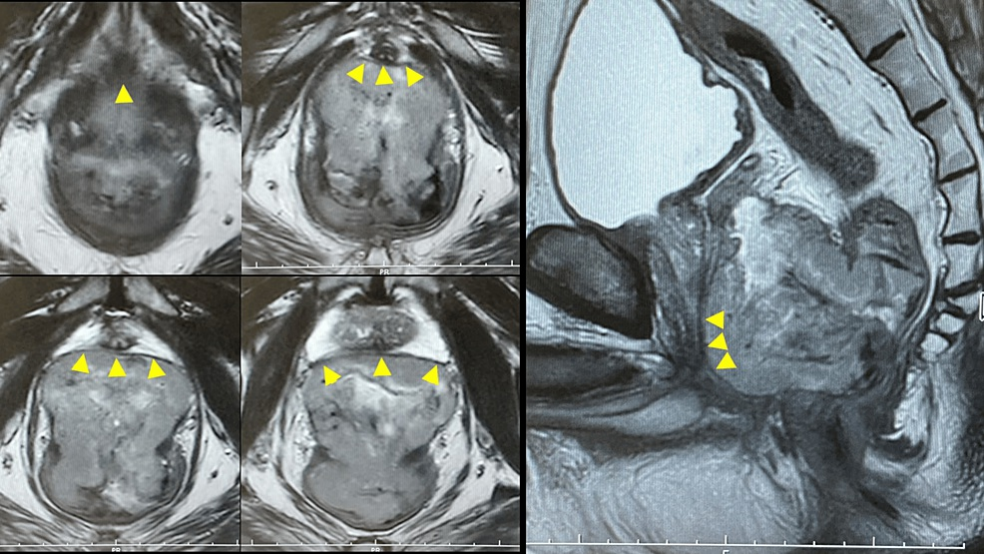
MRI findings prior to the imatinib treatment The MRI showed that a huge rectal GIST was widely in contact with the prostate and urethra (yellow arrows).
